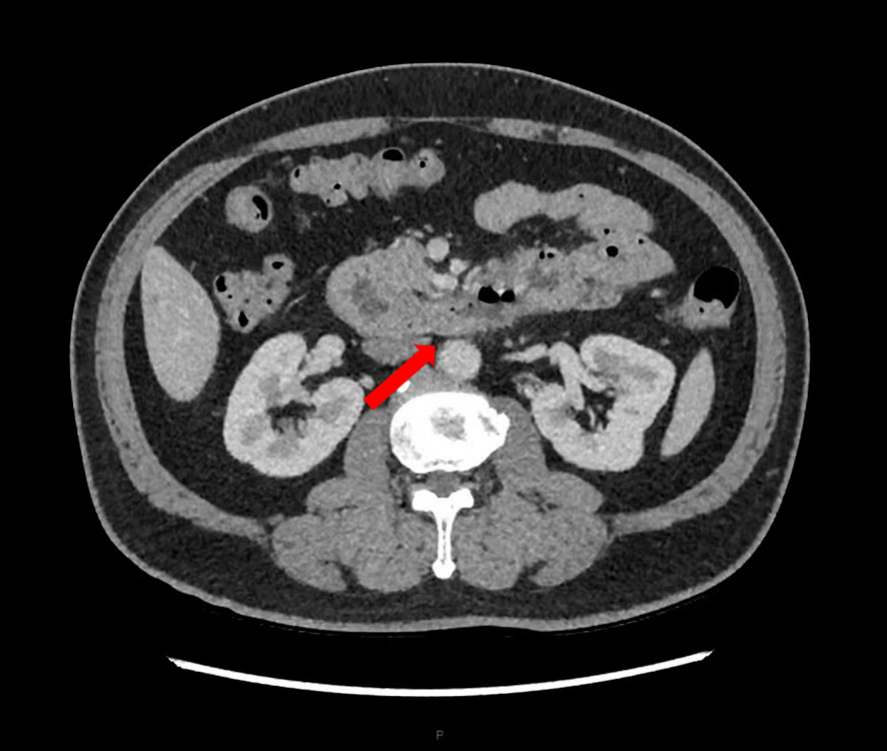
Enhanced abdominal CT revealed thickening and roughness of the horizontal segment wall of duodenum, enlargement and fullness of multiple surrounding lymph nodes, as well as dilatation and effusion in both proximal segments of duodenum and stomach.
